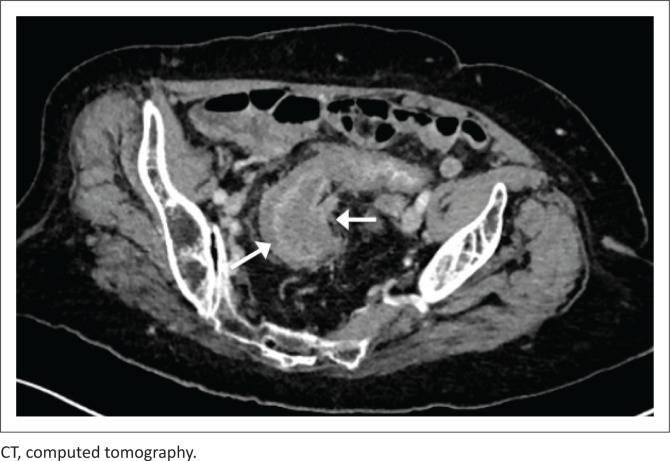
Axial reconstruction of portovenous phase–enhanced CT, demonstrating circumferential mural thickening of the sigmoid colon (arrowed).
